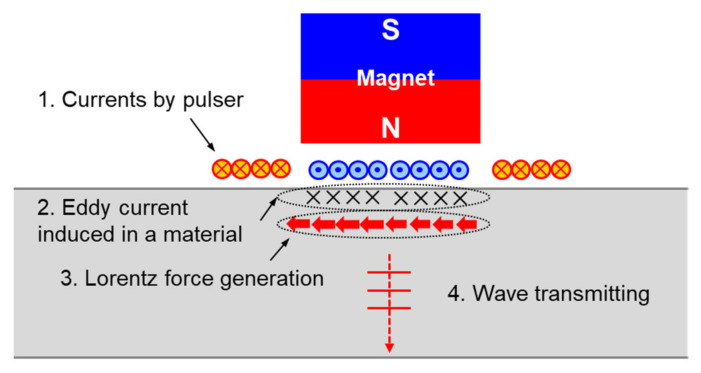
Ultrasonic wave generation mechanism of the EMAT used in the experiments.
